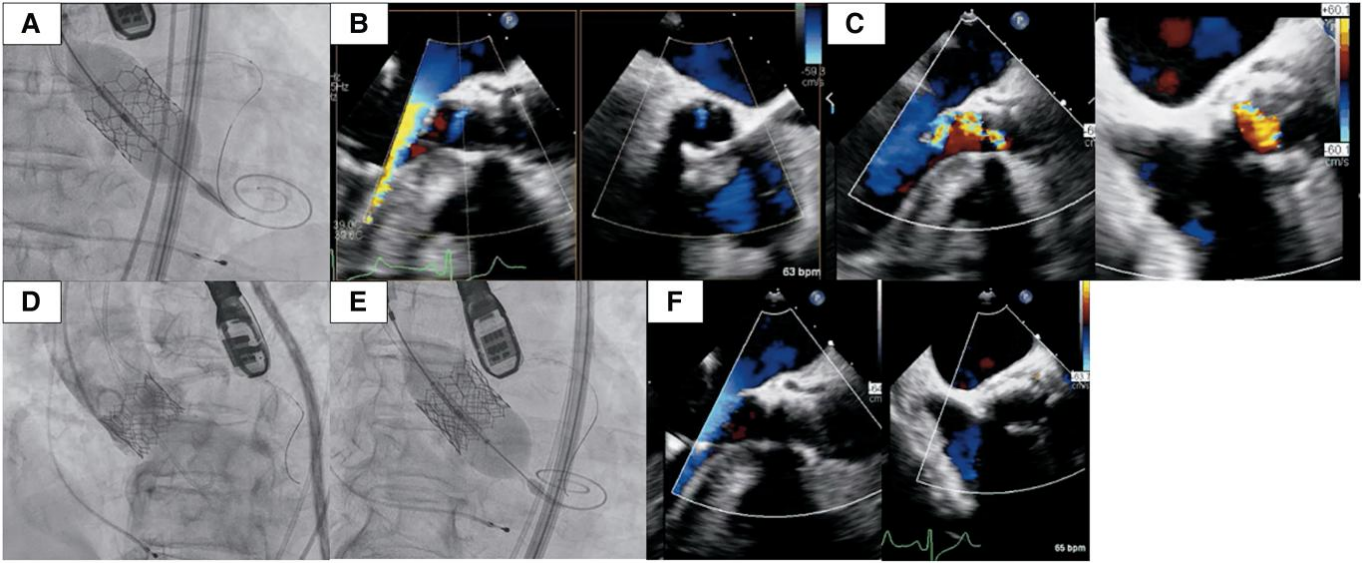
The operative findings during the transcatheter aortic valve implantation procedure are presented. *(A)* Sapien 3 Ultra RESILIA aortic valve with a diameter of 23 mm was deployed. *(B)* Transoesophageal echocardiography showed mild paravalvular leak following deployment. *(C)* Transoesophageal echocardiography demonstrated stuck leaflet and severe intraprosthetic regurgitation. *(D)* Angiography demonstrated Seller’s grade 3 aortic regurgitation. *(E)* Valve-in-valve procedure was performed. *(F)* Final transoesophageal echocardiography showed no paravalvular leak or aortic regurgitation.
